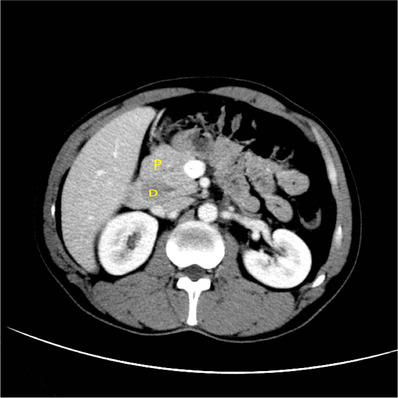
A 39‐year‐old man with abdominal pain. Axial multiple detector spiral computed tomography (MDCT) venous phase shows a triangular configuration of complete annular pancreas. P, pancreas; D, duodenum
